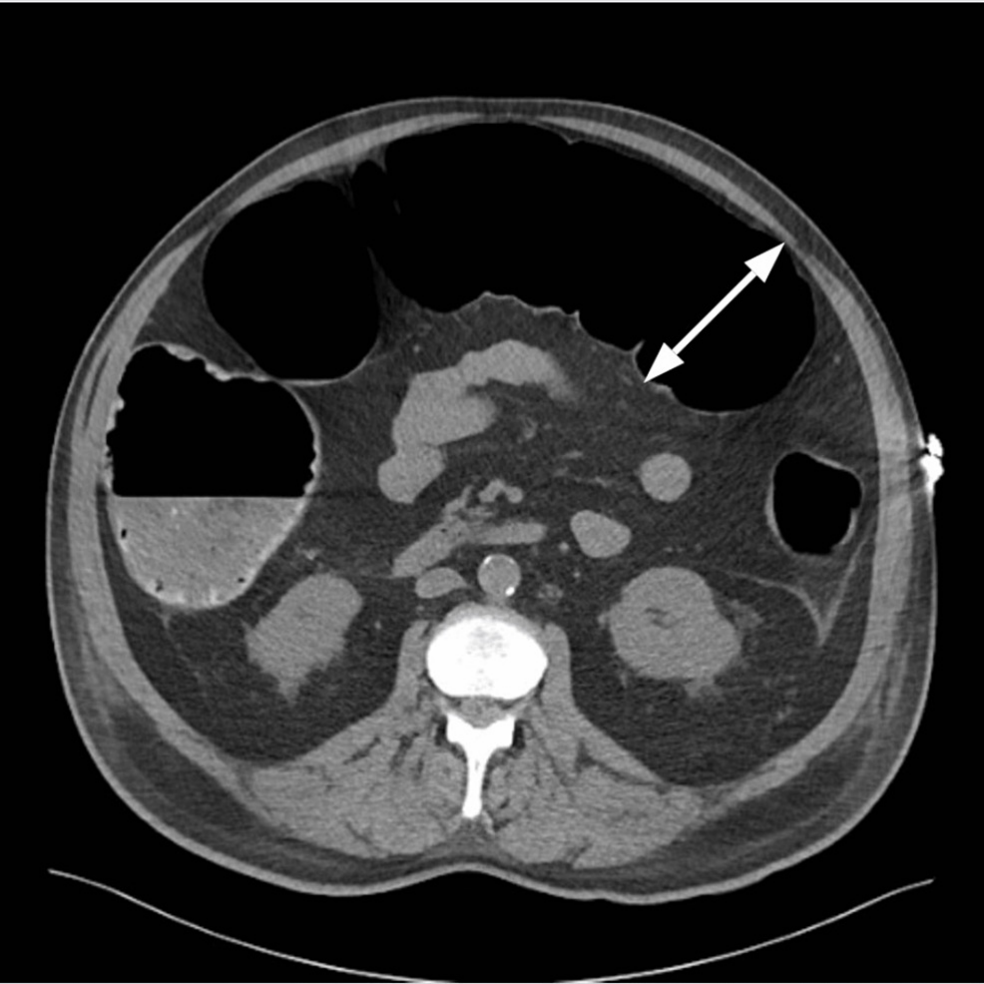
Abdominal CT - transverse view The arrow demonstrates transverse colon distention of around 11 cm CT: computed tomography
